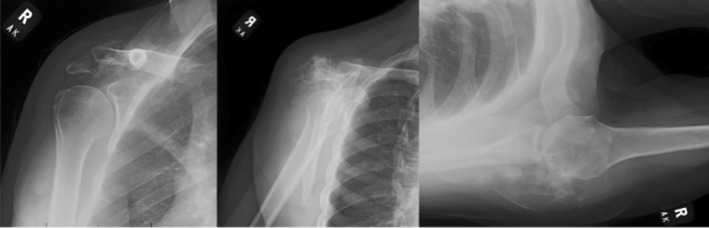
Anteroposterior, scapular‐Y, and axillary radiographs depicting a large heterogeneous mass arising from the right scapula with evident calcifications and bony destruction of the acromion and spine of the scapula which can be seen at the superior aspect of the shoulder joint.
